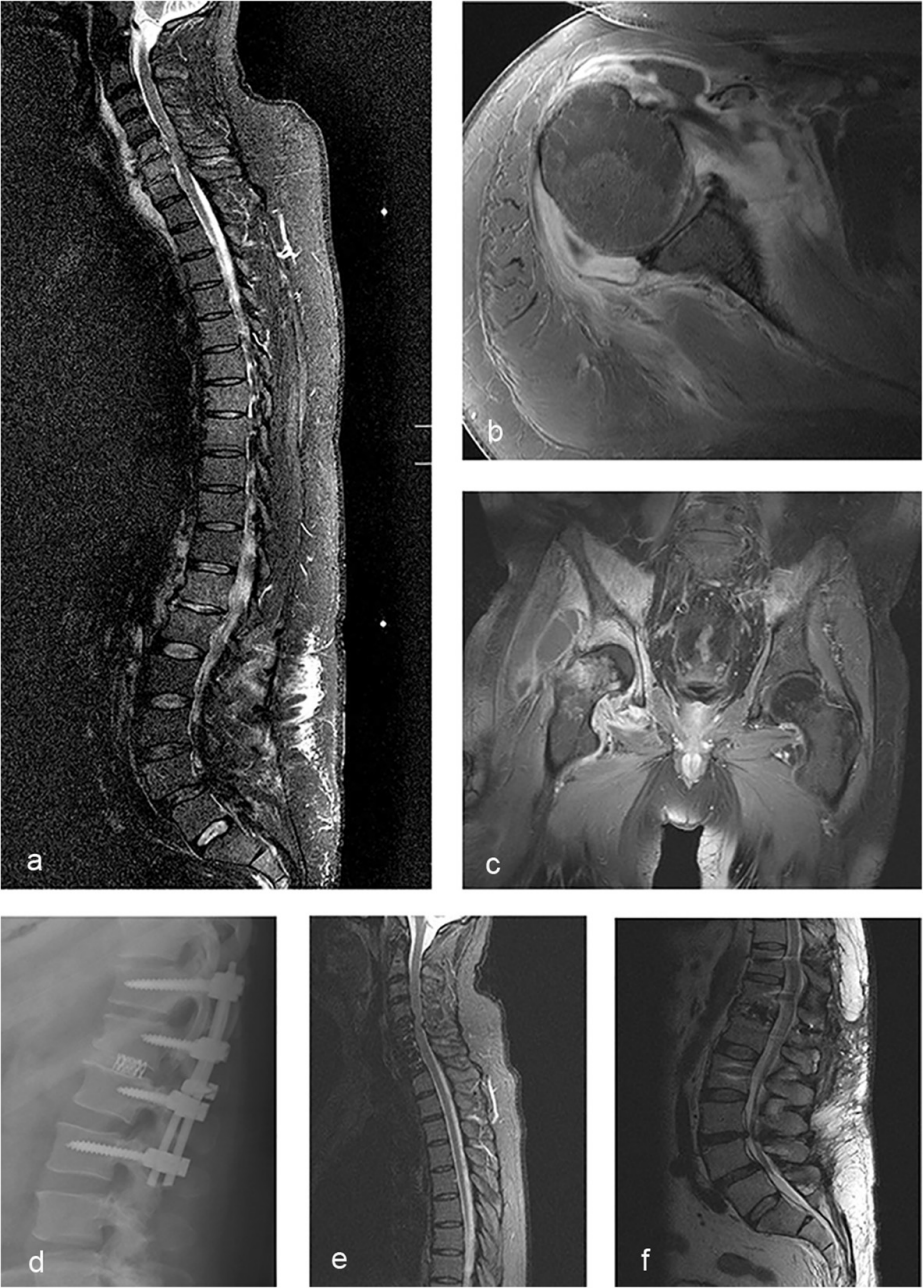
Shows different pictures of one patient: MRI of total spine with spondylodiscitis C5-C7 with a prevertebral and epidural abscess and a spondylodiscitis Th12/L1 (a), bacterial arthritis of the right glenohumeral joint (b), bacterial arthritis of the right hip (c), control x-ray after posterior stabilization Th11-L2 with interbody fusion Th12/L1 (d), follow up MRI after ventral cervical spondylodesis and posterior lumbar stabilization with interbody fusion (e, f).
